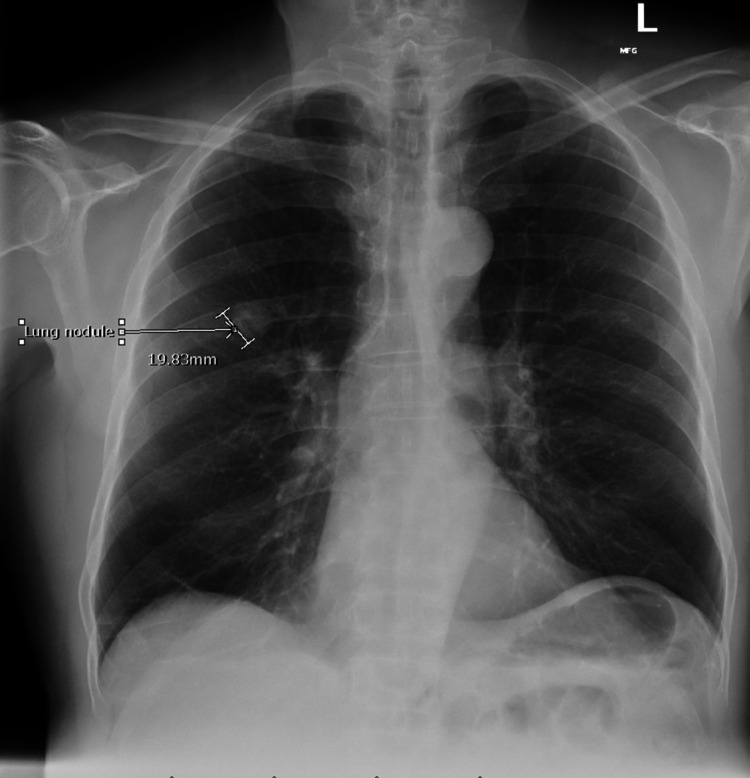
Chest X-ray showing a 2 cm lung nodule
